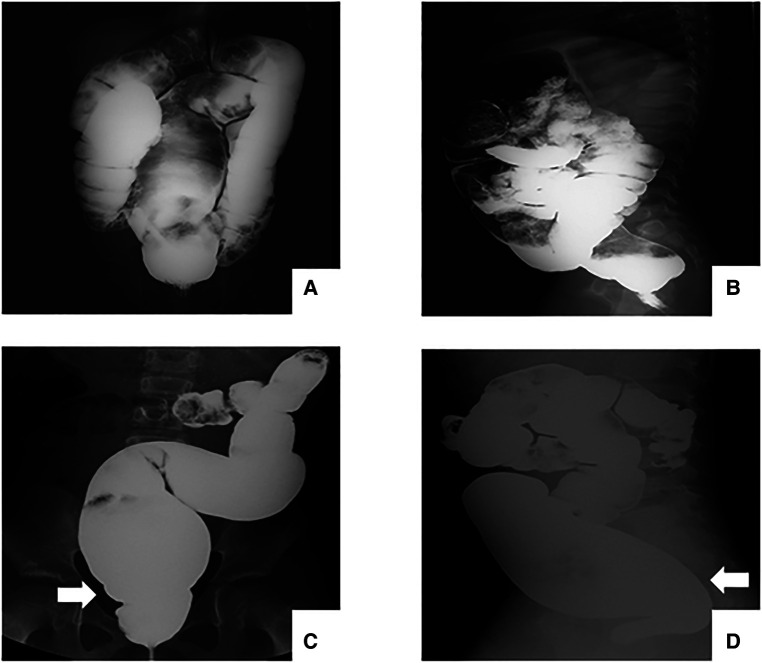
Radiography examination before TEPT. (A-B) patients with USHD shows a suddenly dilated bowel without an obvious transition zone. (C-D) patients with SHD shows a clear transition zone.
